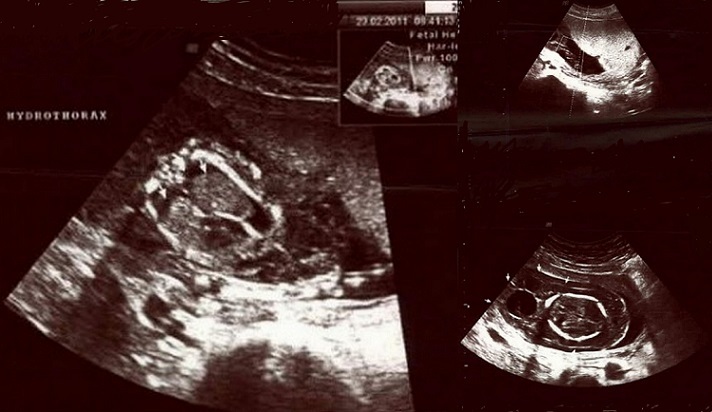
Cystic hygroma, fetal hydrothorax and abnormal umblical artery pulsed Doppler
